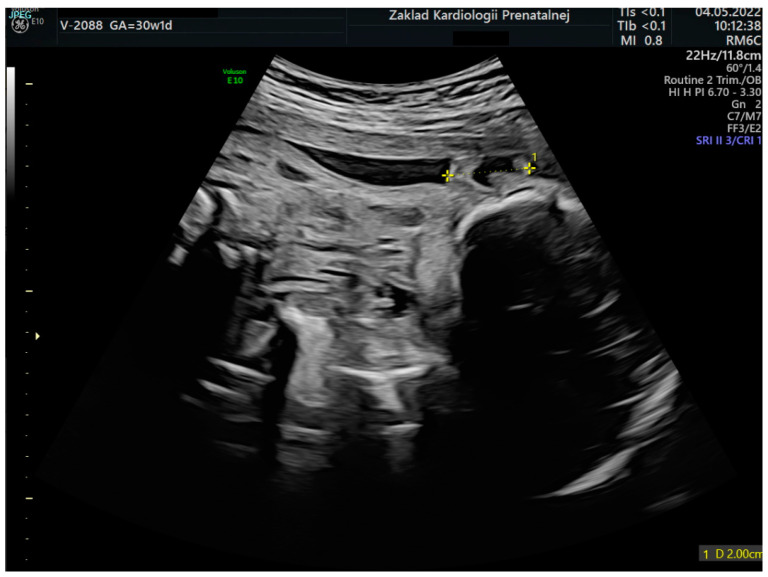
An image of a fetal ear in 2D ultrasound examination. Measurement of the fetal ear at the gestational age of 30 weeks and 1 day.
